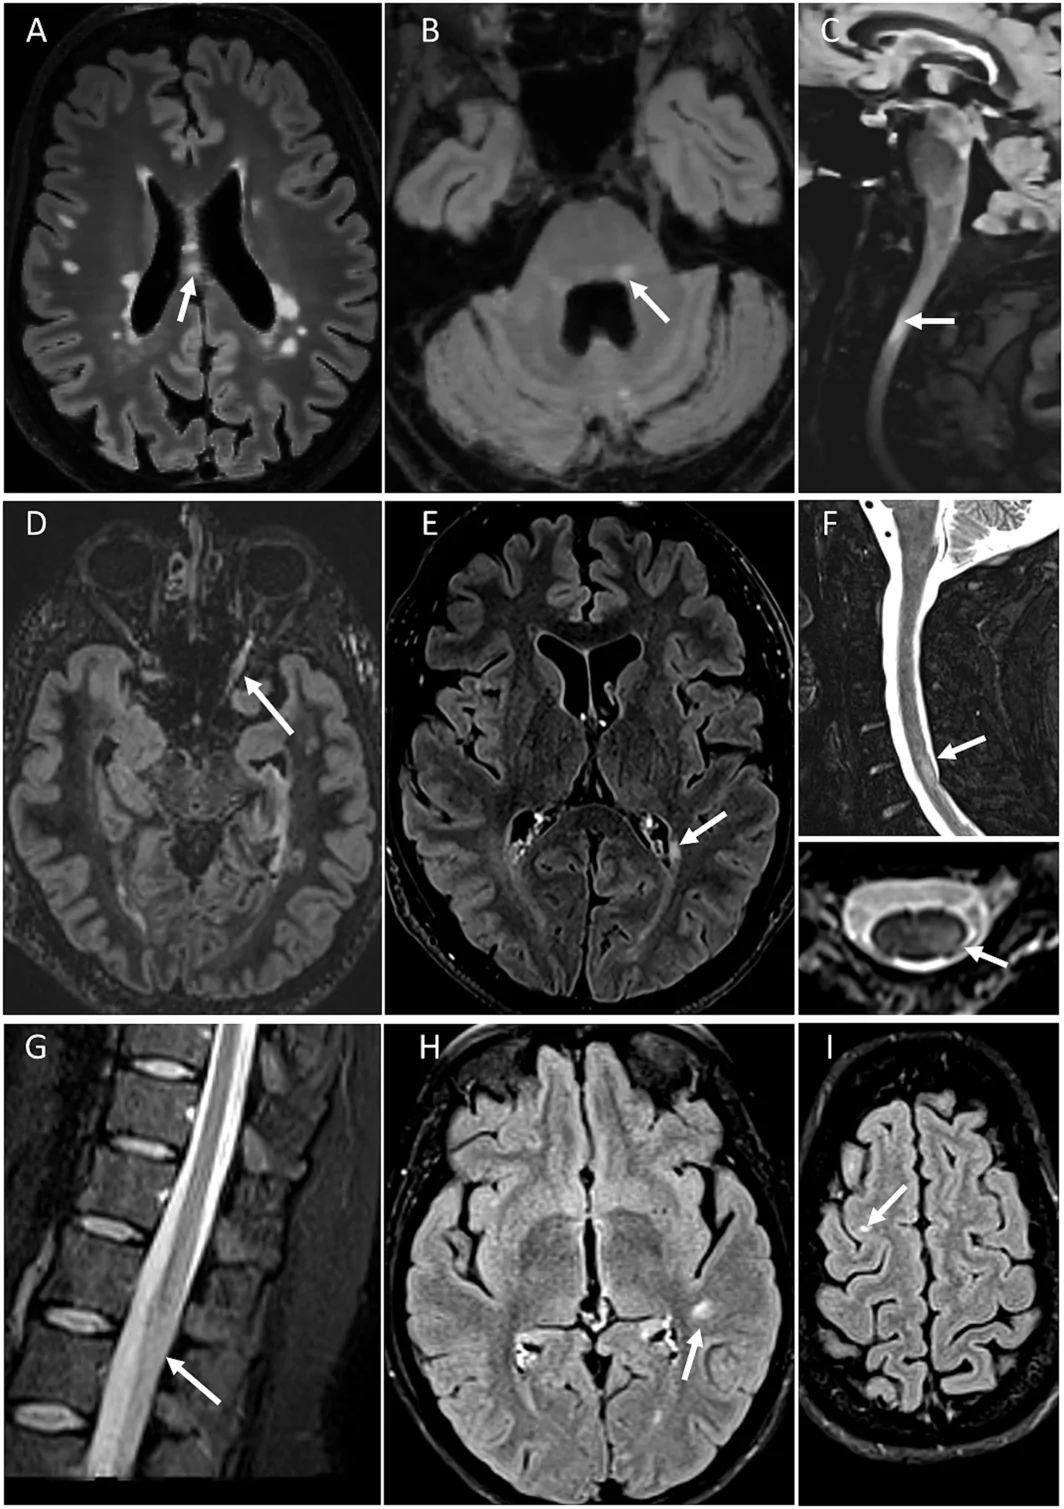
Supporting radiological features in low‐positive patients. Figure 3 shows MRI findings of three low‐positive patients (A‐C, a patient with typical MS lesions; D‐F: A patient with MS and a long optic nerve lesion on brain MRI; G‐I, a patient with MS and a conus lesion on spinal cord MRI).
